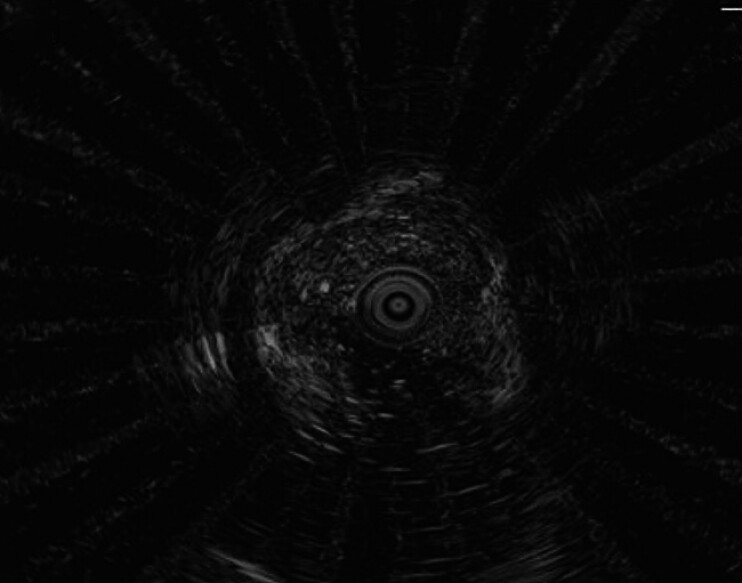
Image during endoscopic ultrasonography showing a slightly hyperintense mass, measuring approximately 122 × 6 mm, originating from the mucosa and muscularis mucosa, along with localized submucosal fibrous hyperplasia.
